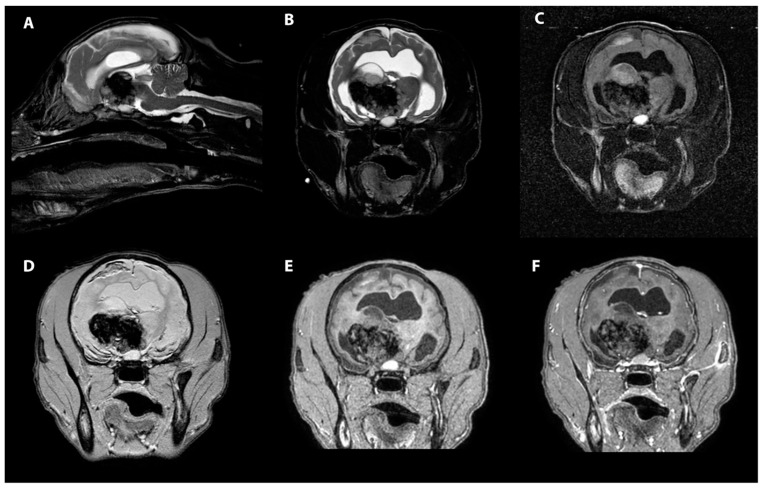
MRI images of the heterogenous intra-axial mass centered in the right thalamus in sagittal T2W (A), transverse T2W (B), transverse T2-FLAIR (C), transverse T2* (D), transverse T1W pre contrast (E) and transverse T1W post contrast images (F). The T1 and T2 hypointensity of the pituitary gland can be seen as well.
